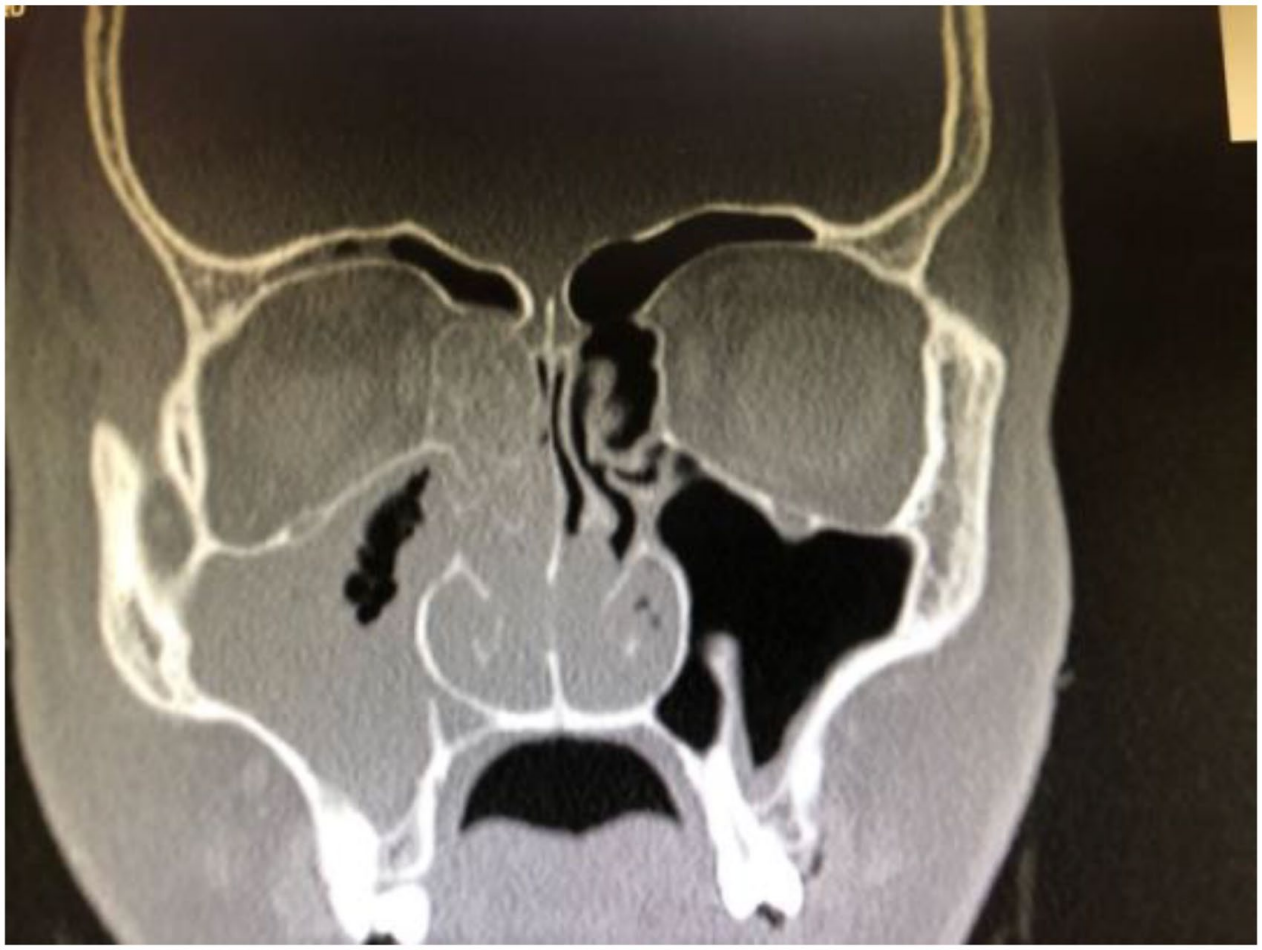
CT scan in sagittal view.
